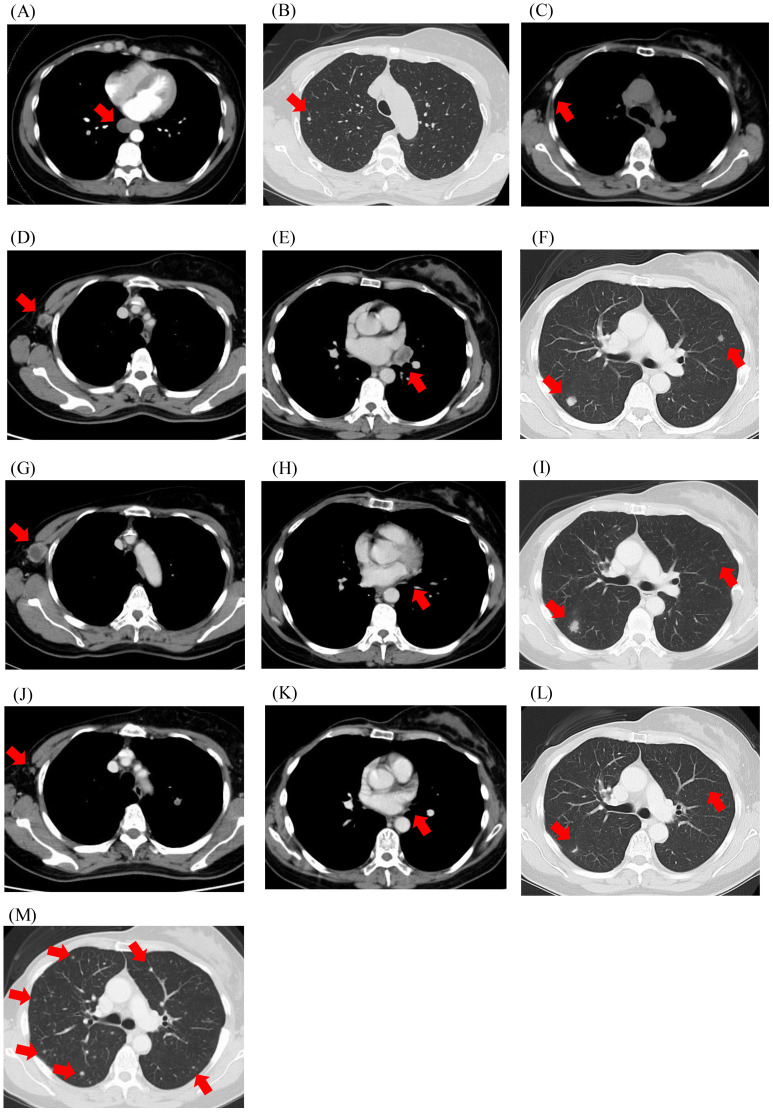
Computed tomography imaging. (A) Upon diagnosis of distant metastasis; (B, C) Before the initiation of chemotherapy; (D-F) Before the initiation of pembrolizumab; (G-I) After two cycles of pembrolizumab; (J-L) After four cycles of pembrolizumab; (M) At disease progression after 10 cycles of pembrolizumab. Red arrows indicate metastatic lesions.
